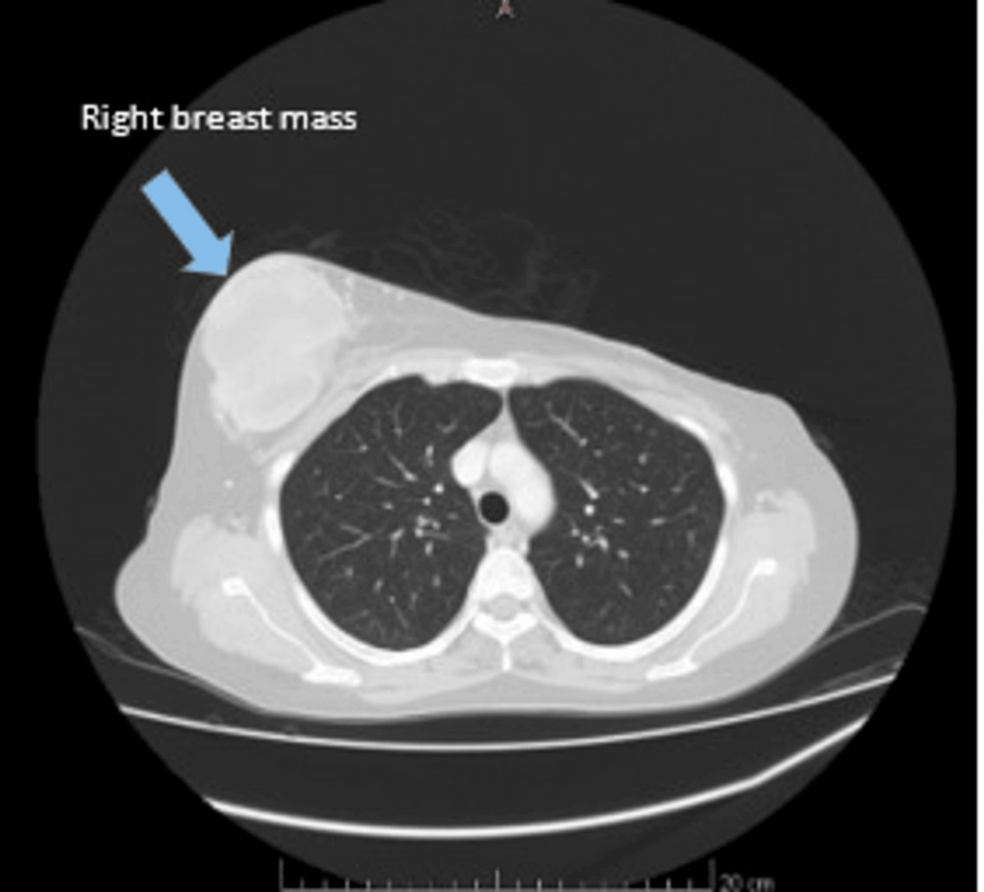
CT imaging (taken July 2020) recurrent swelling in the right breast (blue arrow).
